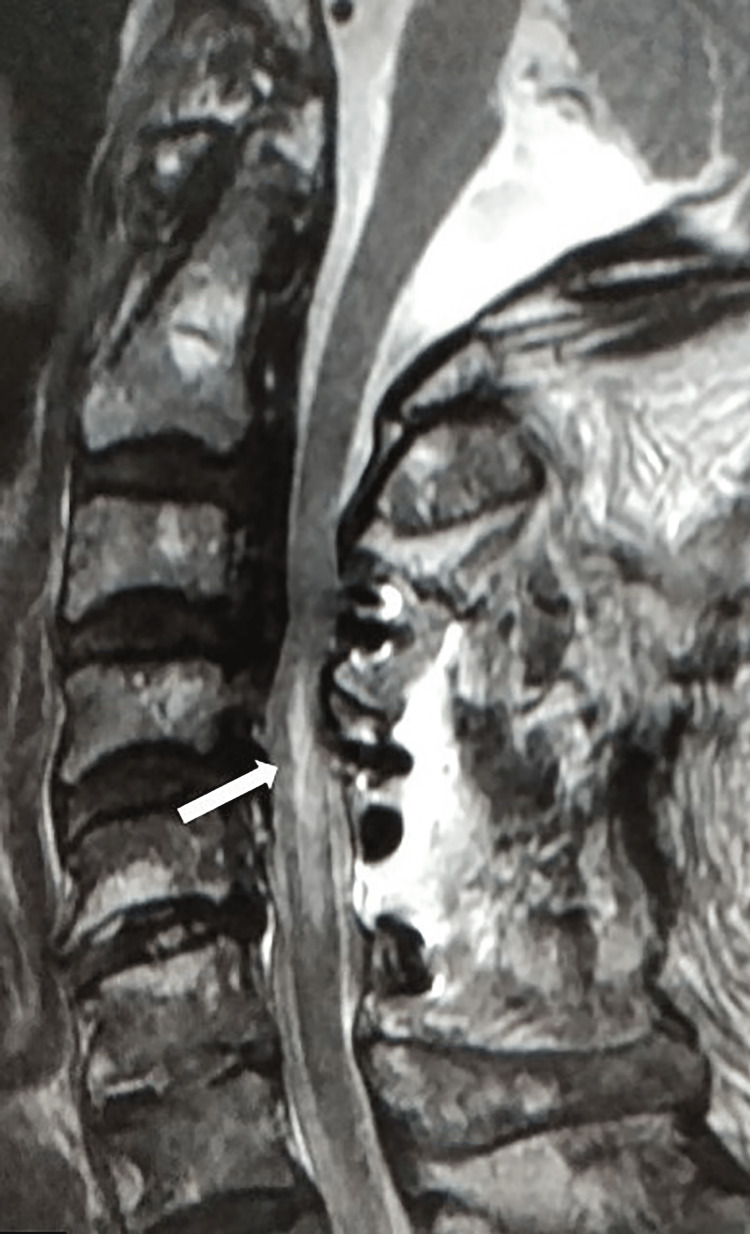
Postoperative sagittal T2-weighted MRI Postoperative MRI disclosed a marked intramedullary T2-high signal intensity at the C4-C5 level (arrow).
